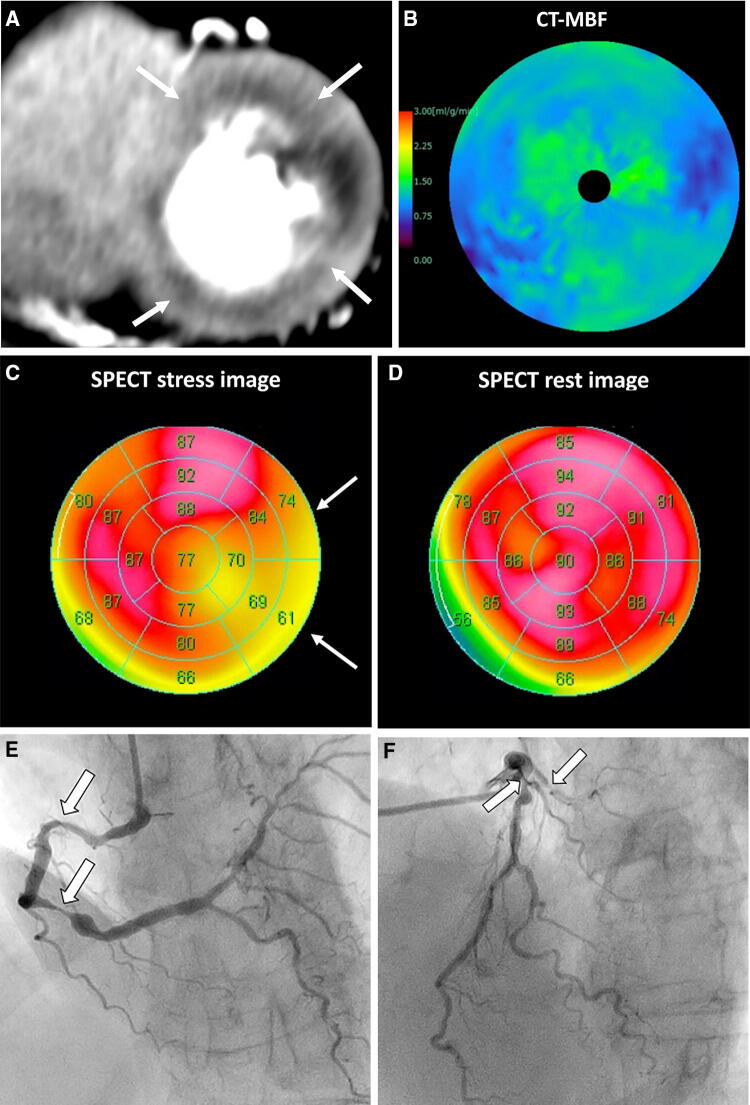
Dynamic CTP scans for triple-vessel disease in an 84-year-old woman with chest pain. a Short-axis view of a dynamic CTP (grayscale) shows a subendocardial perfusion defect in the entire circumference of the heart (white arrows). b A CT-MBF color-coded image shows low CT-MBF throughout the heart. SPECT during c stress and d at rest shows a reversible perfusion defect in the lateral wall but no marked perfusion defect in the other regions. Invasive coronary angiography of the e right and f left coronary arteries shows severe triple-vessel disease. In this case, single-photon emission tomography did not accurately detect the presence of triple-vessel disease, known as balanced ischemia. *CTP* computed tomography perfusion, *CT-MBF* computed tomography derived-myocardial blood flow, *SPECT* single-photon emission computed tomography
